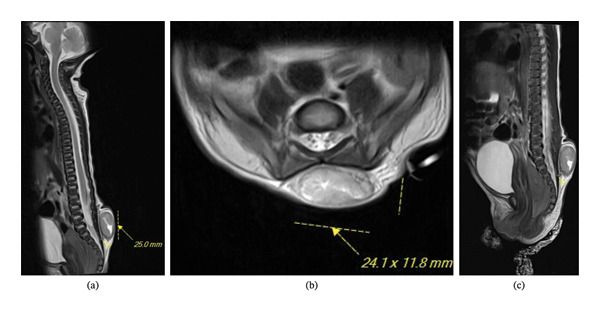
MRI of a 1‐day‐old female. (a) Sagittal T2 image demonstrating a tethered conus medullaris terminating at L3 with an intradural juxtamedullary (subpial) conus lipoma extending dorsal (L2) and caudal (L5) and measuring up to 0.3 cm in axial diameter. (b) Axial image depicting a 2.4 × 1.2 cm axial (2.5 cm craniocaudal) transmidline circumscribed mass between superficial cutaneous tissue and superficial myofascial plane spanning from L4 to S3. (c) Sagittal T2 image demonstrating potential sinus tract extending through dorsal margin of canal at S3 upper aspect.
